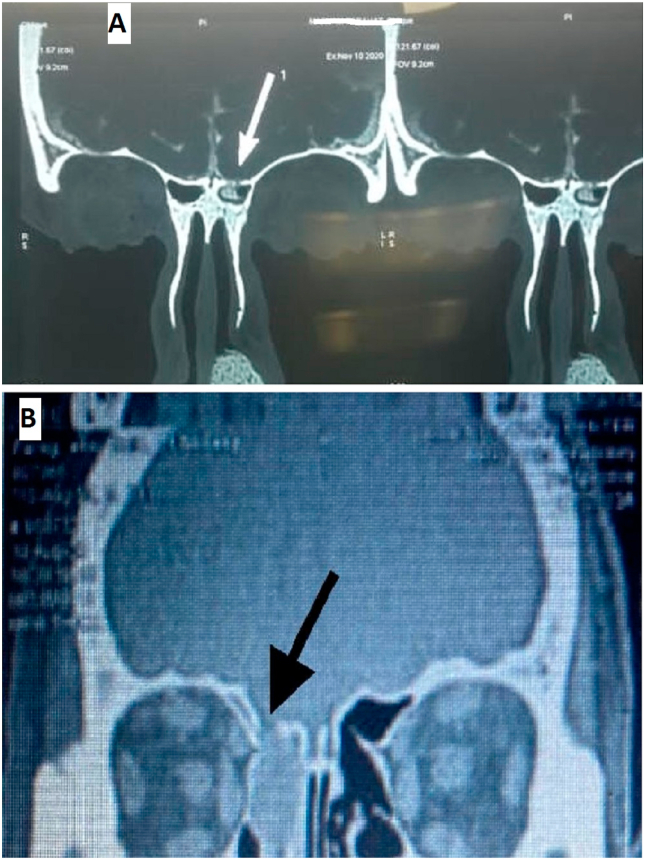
CT scan coronal cuts pictures; A) Nose and paranasal sinus showing frontal sinus recess defect. B) Nose and paranasal sinus showing a defect in the medial part of frontal sinus posterior table.
